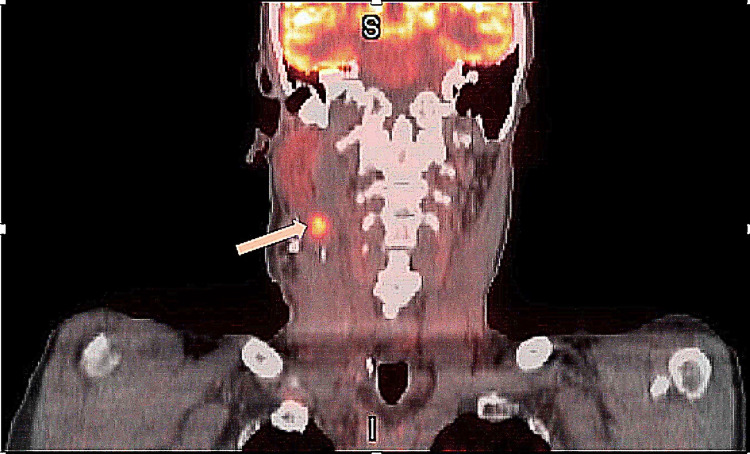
Coronal view PET scan showing increased fluorodeoxyglucose (FDG) uptake in the right level 2B (upper jugular) lymph nodes (arrow).
